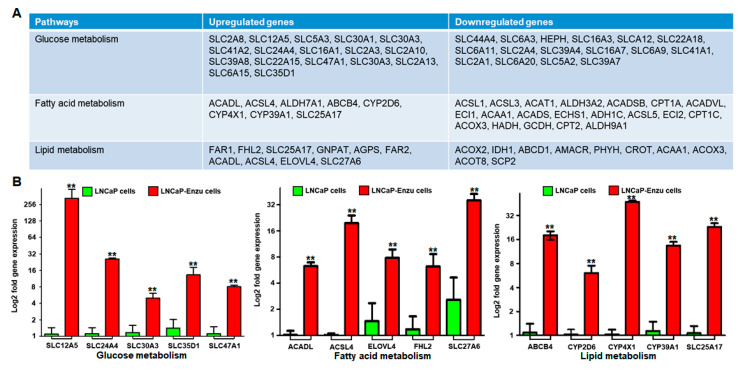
(A) Alteration in gene expression associated with the regulation of glucose, fatty acid, and lipid metabolism in LNCaP (parental) and LNCaP enzalutamide resistant cells. (B) Real time PCR validation of genes of glucose metabolism *SLC12A5*, *SLC24A4*, *SLC30A3*, *SLC35D1*, and *SLC47A1*; fatty acid metabolism *ABCB4*, *CYPD6*, *CYP4X1*, *CYP39A1*, and *SLC25A17*; and lipid metabolism *ACADL*, *ACSL4*, *ELOVL4*, *FHL2*, and *SLC27A6*. The qRT-PCR data were analyzed between LNCaP versus LNCaP-enzalutamide resistant cells using (delta-delta CT method; fold = 2^−ΔΔCT^). Bar represents the standard error mean (SEM) for three biologicals and three technical replicates.  *p* < 0.001, LNCaP versus LNCaP enzalutamide resistant cells.
